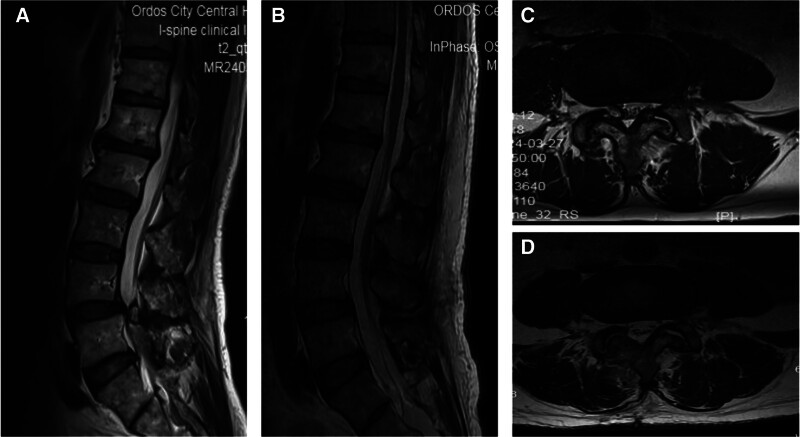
Preoperative and postoperative lumbar spine MRI. (A and C) Sagittal and axial T2-weighted imaging shows a round signal shadow on the right side of the spinal canal at the L4/5 level. (B and D) Three months after surgery, sagittal and axial T2-weighted MRI of the lumbar spine showed that the intraspinal mass at the L4/5 level was completely removed without recurrence. MRI = magnetic resonance imaging.
